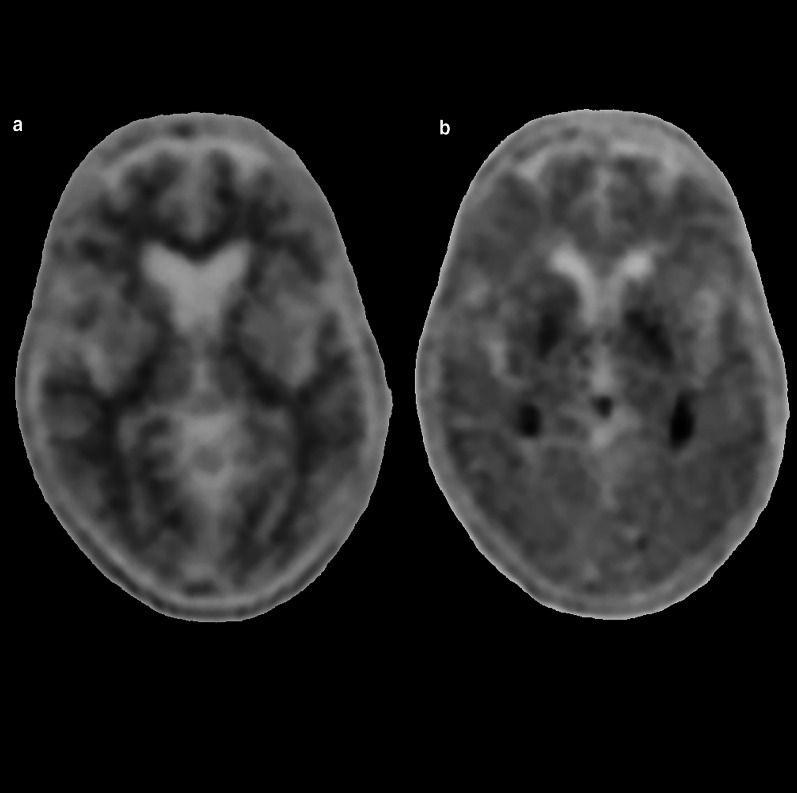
A 65-year-old female with reported gradual decline in short term memory and depressive symptoms, initial MoCA 25/30. Florbetapir PET/CT demonstrates normal white matter distribution with no regions of abnormal cortical uptake, compatible with no to sparse amyloid plaque (a). Flortaucipir PET/CT demonstrates no cortical tau retention with off-target binding in the bilateral choroid plexuses and basal ganglia (b). Score on MoCA improved to 29/30 on antidepressant therapy. Final diagnosis was pseudodementia related to major depressive disorder
